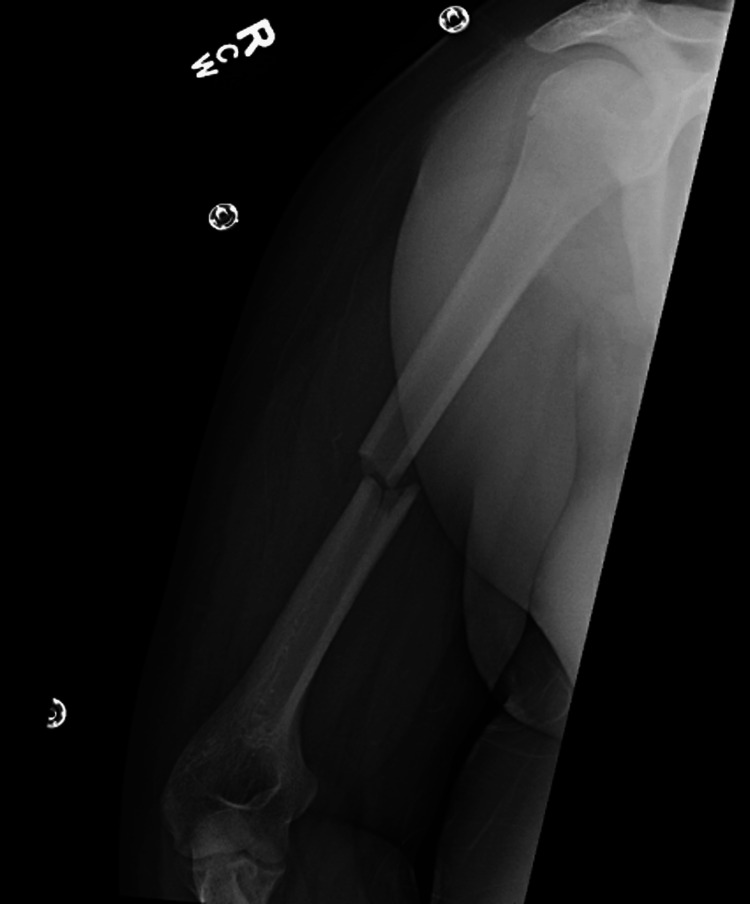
AP radiograph of the right humerus taken on the day of the injury in the emergency room. The image shows a transverse fracture of the middle humeral shaft with minimal comminution.
